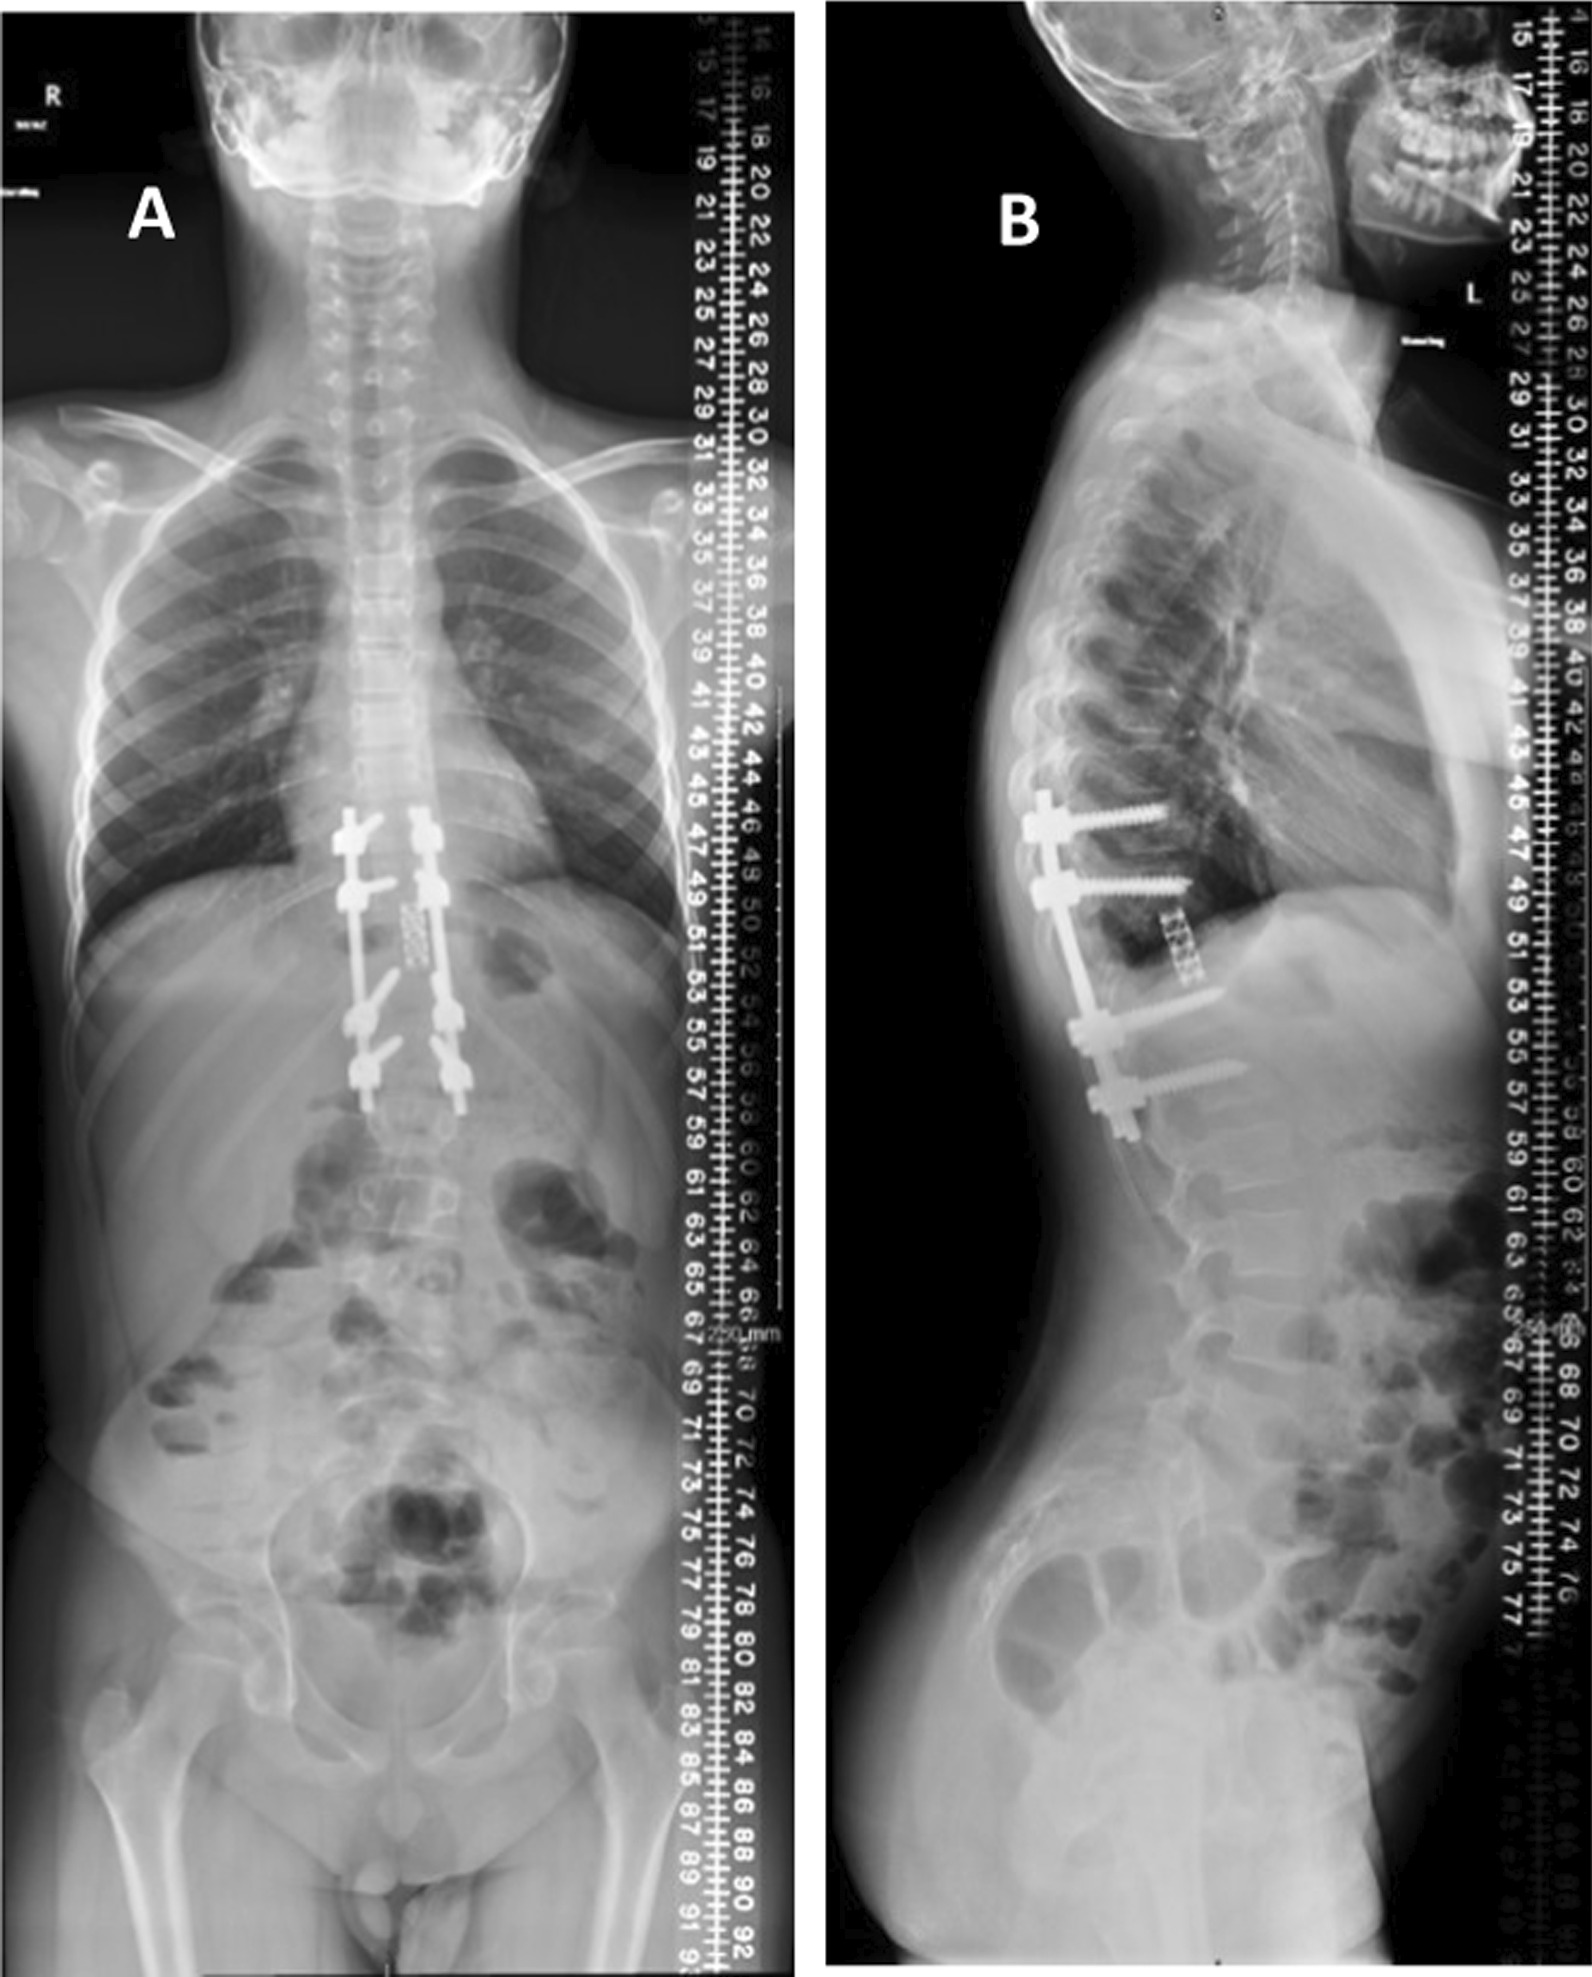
A, B scoliosis X-ray A anteroposterior (AP) and B lateral, 4 months post first surgery showing T11 resected vertebral body with cage placement, thoracolumbar fixation rods and screws, with a scoliosis measure of 6.5°
